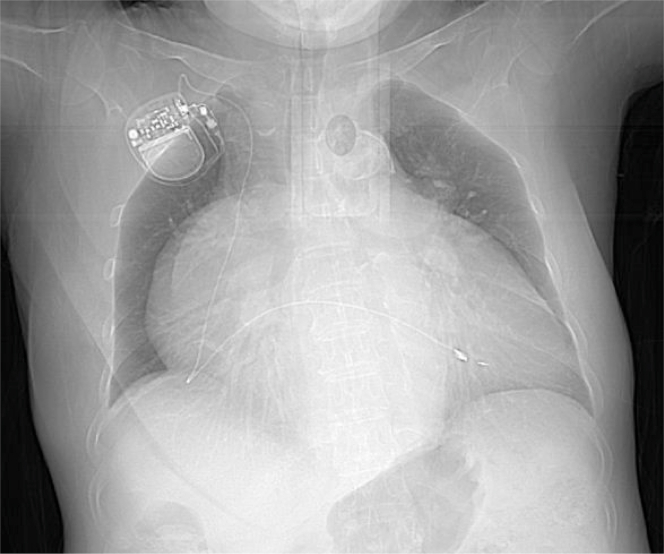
Chest Radiograph The chest radiograph shows that the patient has a huge heart.
